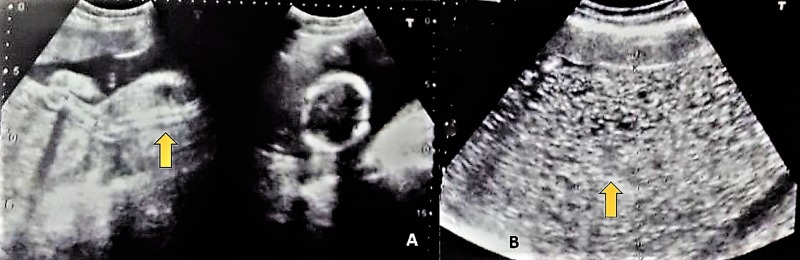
Ultrasound scans indicative of partial mole with a living fetus. A indicates enlarged placenta. B indicates partial mole with multiple cystic spaces in the placenta in a “snowstorm pattern.”
